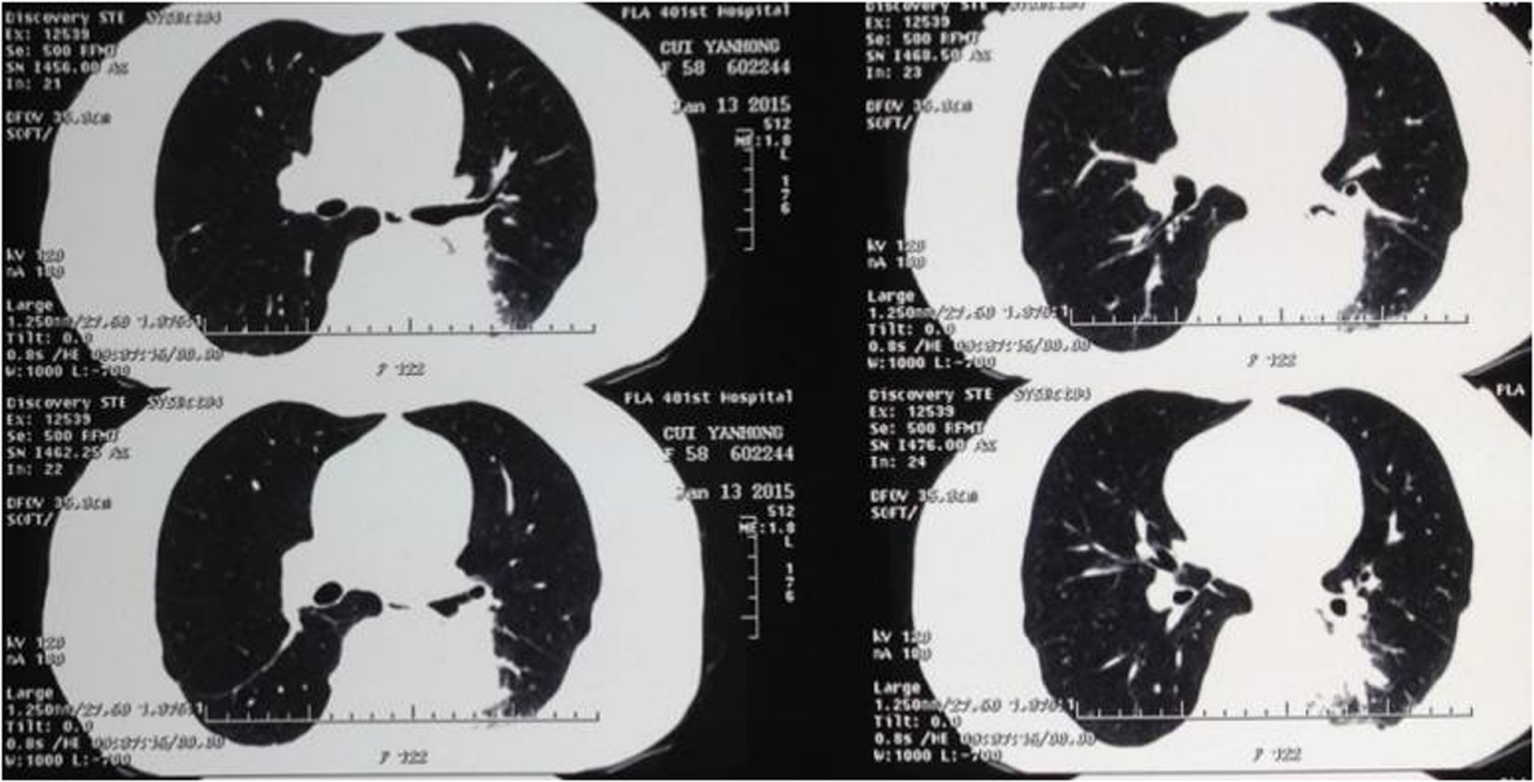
CT scan revealed a mass located at left lower lobe and the main and upper lobe bronchus were not involved
